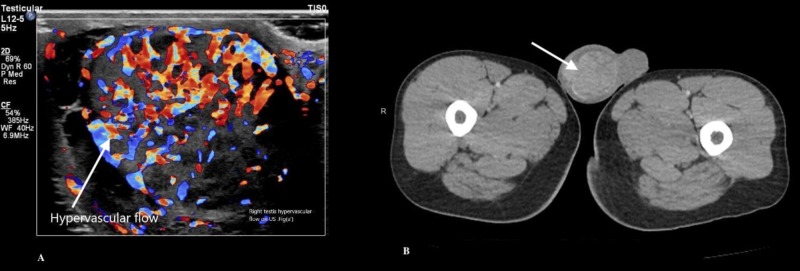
Testicular US Doppler (A) and CT scan of pelvis (B) showing heterogenous texture, multiple hypoechoic masses, with septations and hypervascular flow in the right testis US: ultrasound; CT: computed tomography
